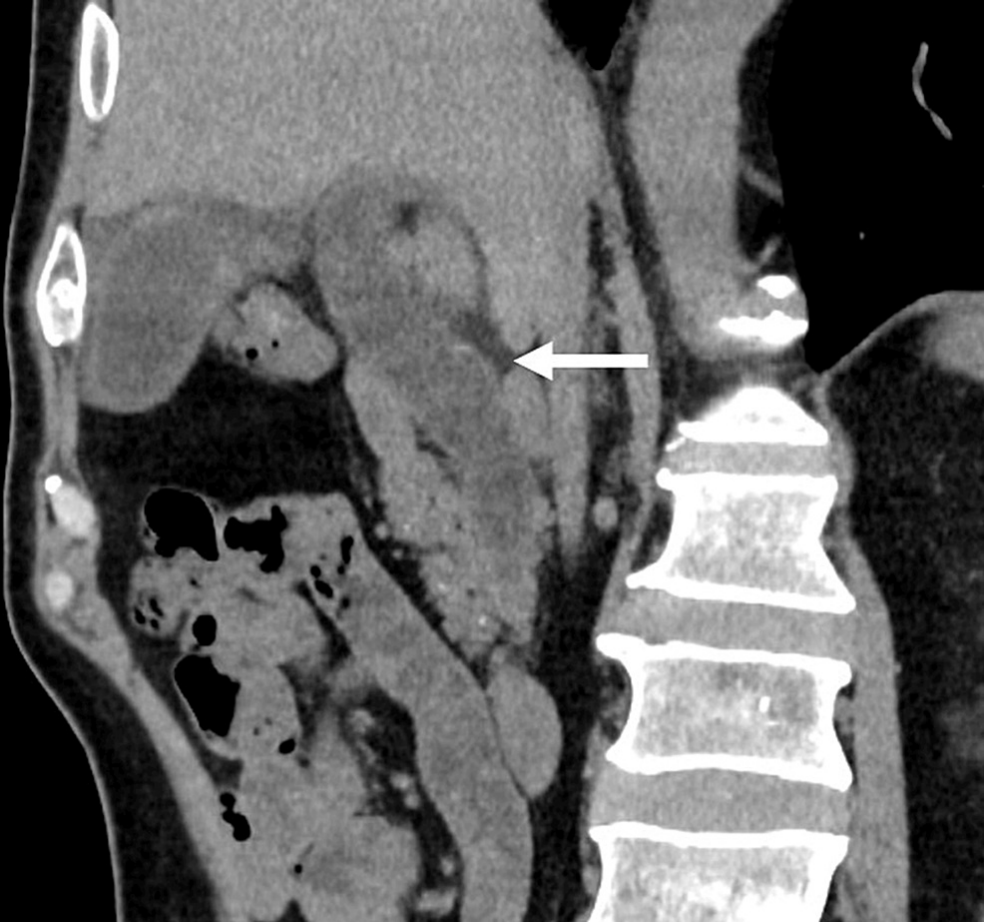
Abdominal computed tomography on admission. Remarkable dilatation and wall thickening of the extrahepatic bile duct (arrow) were noted.
